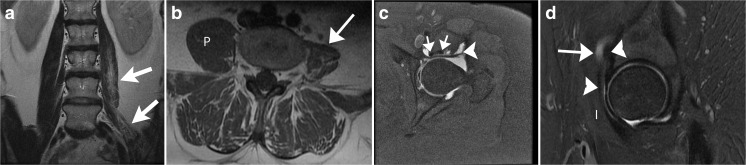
A 40-year-old woman underwent lumbar spine MRI for evaluation of left lower extremity weakness. The psoas findings were unexpected and prompted a medical records search, confirming previous psoas release at the time of hip arthroscopy, and increasing weakness dating from that surgery. There were no spinal abnormalities to cause the psoas atrophy. Hip MR arthrogram was subsequently performed. a Coronal T2 MRI at 1.5 T shows marked atrophy of the left psoas muscle (*arrows*) relative to the right. The muscle is small, and streaks of fat are interspersed with the muscle fibers. b Axial T1-weighted MRI at 1.5 T shows that the left psoas (*arrow*) is smaller than the right, and contains high signal intensity fatty streaks consistent with atrophy.* P* normal right psoas muscle. c Axial T1FS MR arthrogram at 1.5 T shows the surgically split psoas tendon (*arrows*). Also note the anterior joint capsule defect (*arrowhead*); this patient did not have instability symptoms. d Sagittal PDFS MR arthrogram at 1.5 T shows fluid (*arrow*) tracking along the psoas tendon (*arrowheads*). The iliacus (*I*), anterior to the psoas tendon, appears normal
